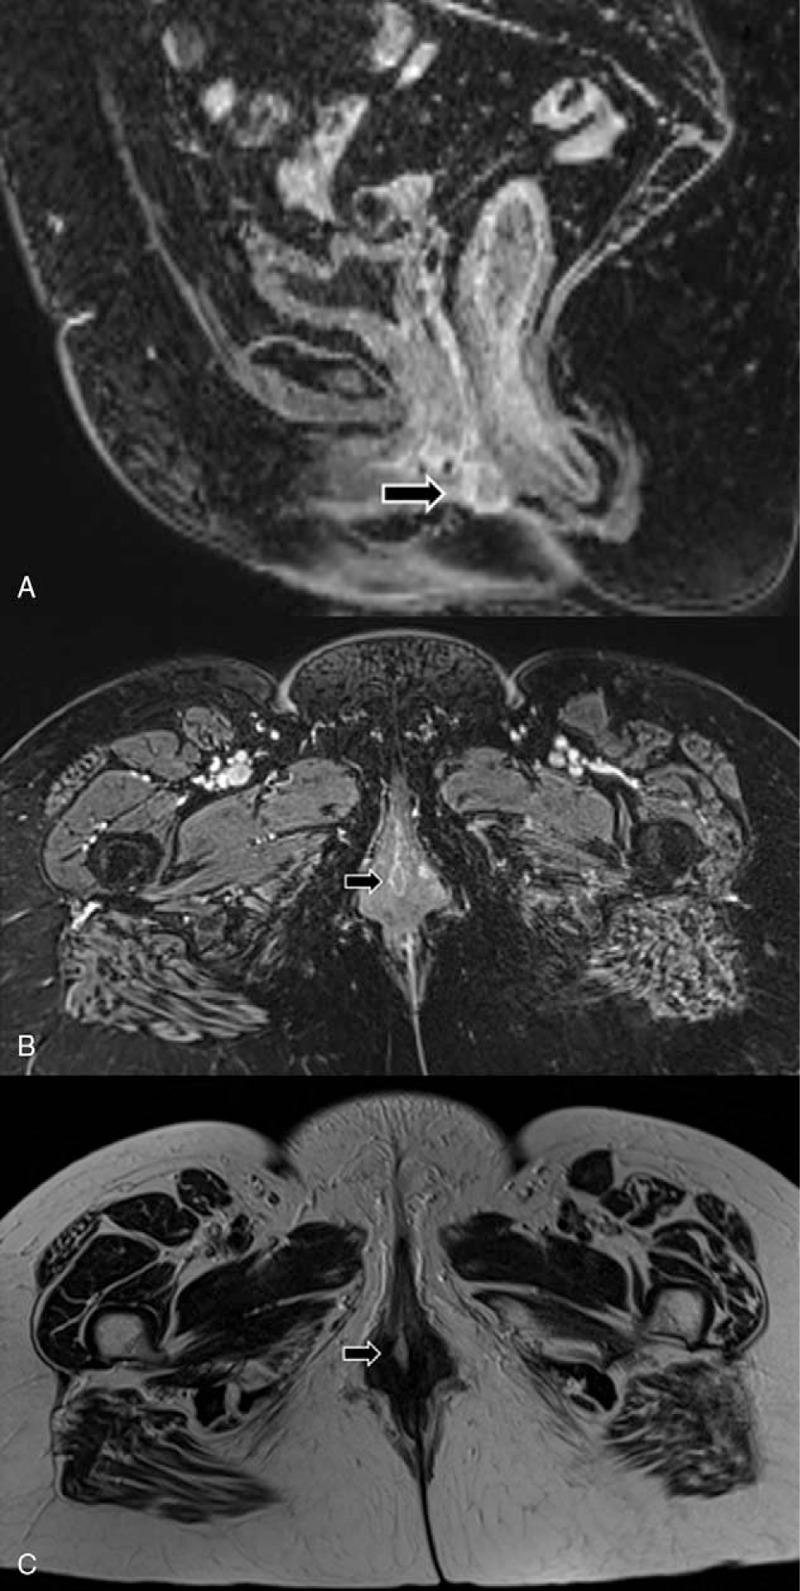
Magnetic resonance imaging (MRI). A, Sagittal contrast-enhanced T1-weighted MRI scan shows an enhancing mass (arrow) in vulva. B, Axial contrast-enhanced T1-weighted MRI scan shows enhancing mass (arrow) in right vulva. C, Axial T2-weighted MRI scan shows hyperintense mass (arrow) in right vulva.
